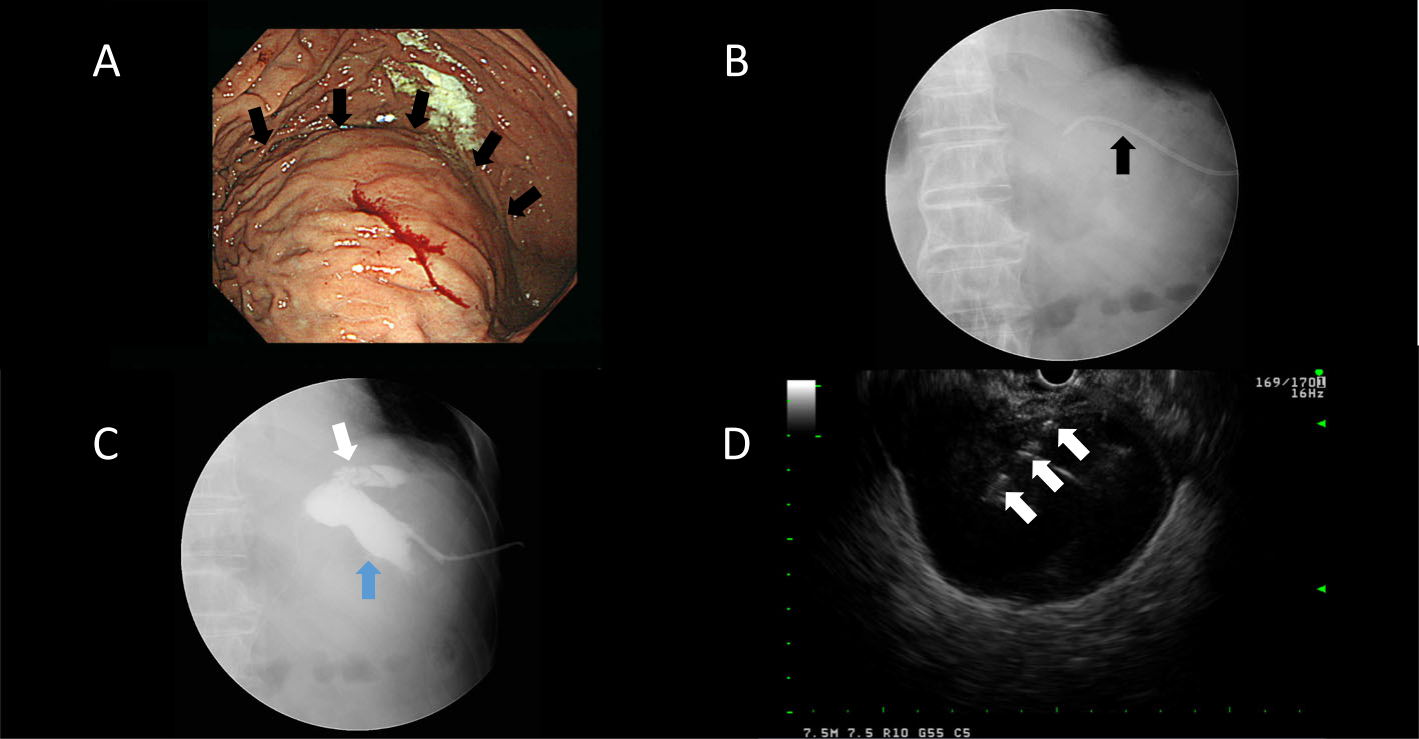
Endoscopic and endoscopic ultrasound findings. a An elevated lesion similar to a submucosal tumor was observed at the greater curvature of the stomach (arrows). b An 8 Fr catheter was placed in the intra-abdominal abscess before fistulography. c Fistulography showed connection between the intra-abdominal abscess (white arrow) and gastric submucosal abscess (blue arrow). Size of gastric submucosal abscess was larger than that of intra-abdominal abscess. d The gastric submucosal abscess was accessed under guidance using a 19-gauge fine-needle aspiration needle (arrows)
